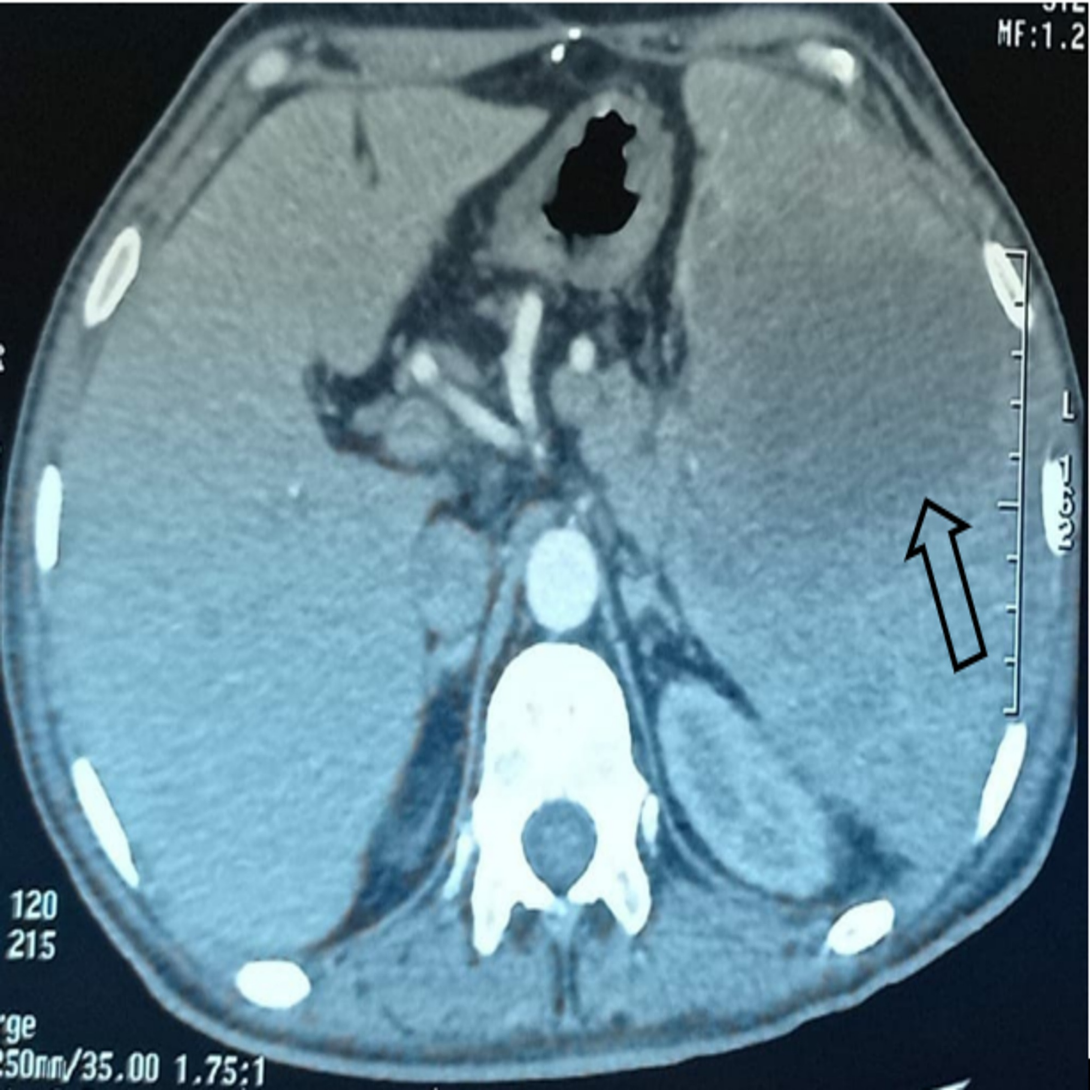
Computed tomography scan showing hypodense areas within the spleen (black arrow)
